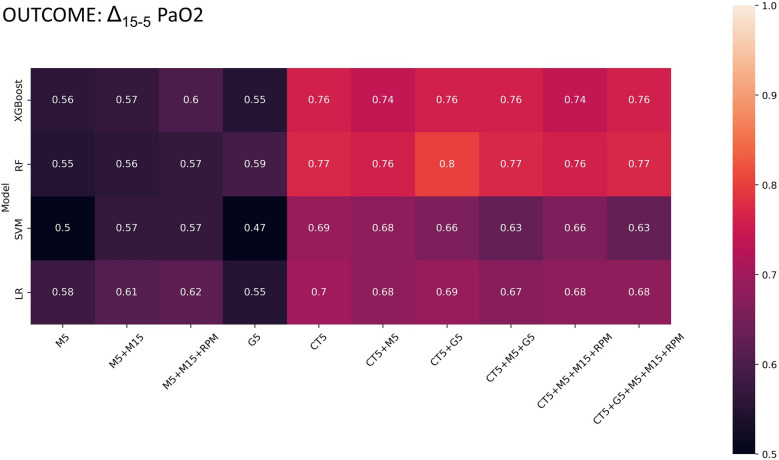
Validation AUC for each pair of dataset and machine learning algorithm, when lung recruitability was gas exchange-defined (recruiters: Δ_15-5_PaO_2_ > 24 mmHg). M5, lung mechanics at PEEP 5 cmH_2_O, M15, lung mechanics at PEEP 15 cmH_2_O, RPM, respiratory partitioned mechanics, G5, gas exchange measured at PEEP 5 cmH_2_O, CT5, CT imaging acquired at PEEP 5 cmH_2_O. XGBoost, gradient-boosted tree; RF, random forest; LR, logistic regression; SVM, support vector machine
